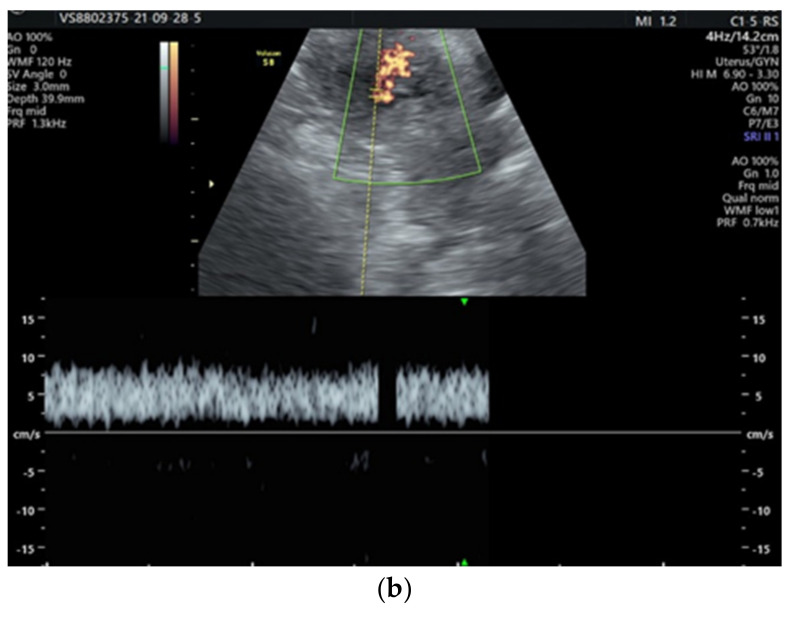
Pulsatile Doppler ultrasound: (a) Arterial flow; (b) venous flow.
